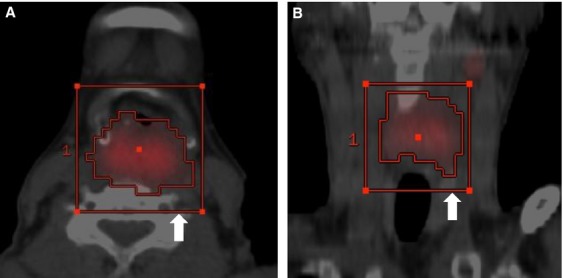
Measuring PET/CT parameters. Pretreatment transaxial (A) and coronal (B) PET/CT images of 64-year-old male with cT4aN2c hypopharyngeal cancer. The region of interest (a rectangular parallelepiped; white arrow) was set to include the primary tumor, and the SUV_max_, SUV_mean_, metabolic tumor volume (MTV), and total lesion glycolysis (TLG) were calculated automatically with the set of SUV threshold. In this case, PET/CT parameters were as follows: SUV_max_ = 15.5, SUV_mean_ = 6.0, MTV = 40.9 mL, and TLG = 245.5 g. SUV, standardized uptake value.
